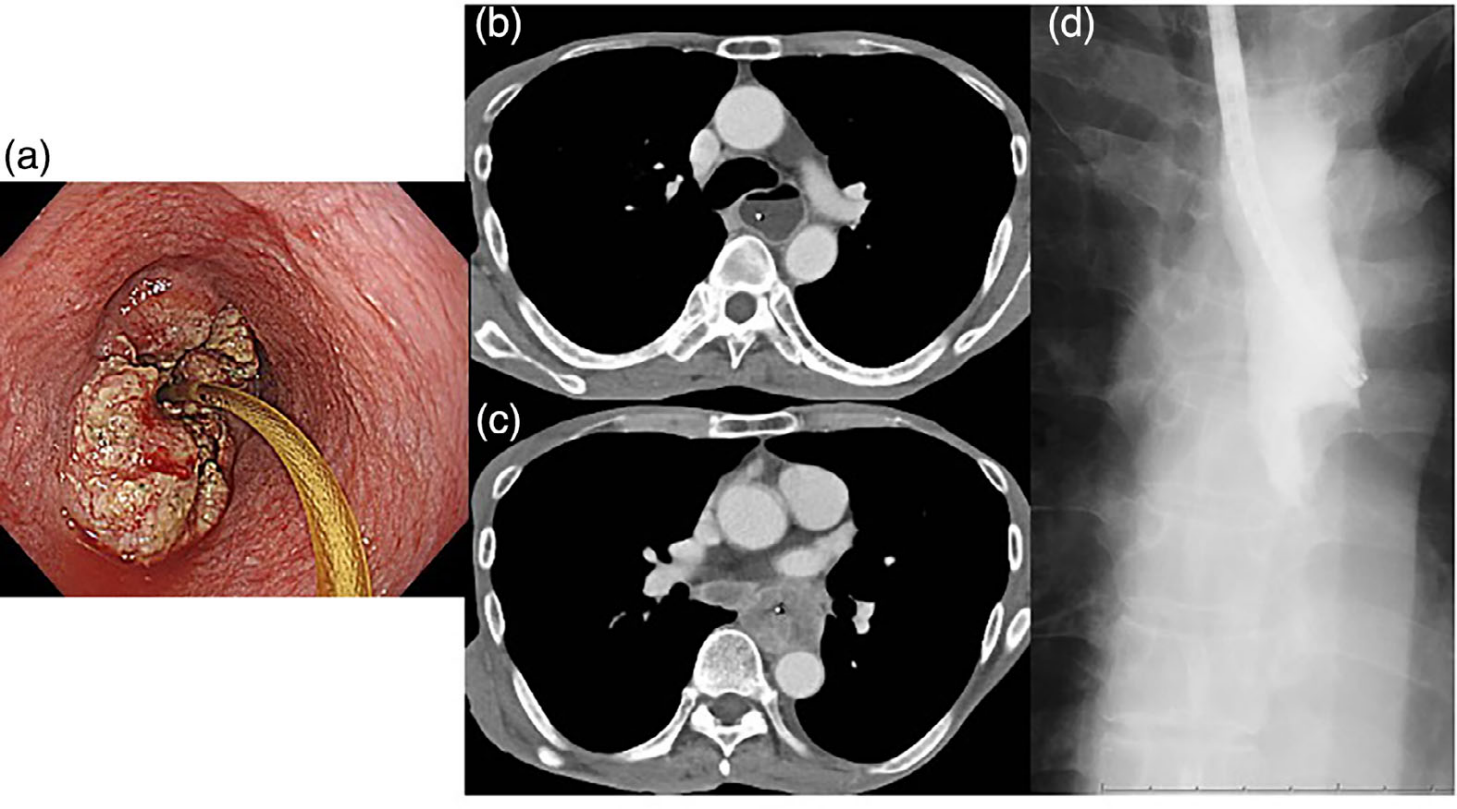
Endoscopy, computed tomography, and radiographic findings in Case 1. (a) Tumor has a severe stricture which the narrow nasogastric tube can pass through. (b) Computed tomography shows the dilatation of the esophagus at the proximal side of the malignant stricture. (c) Computed tomography shows the esophageal tumor with lymph node enlargement. (d) The ultrathin endoscope cannot pass through the stricture, and Gastrografin pooling can be observed.
